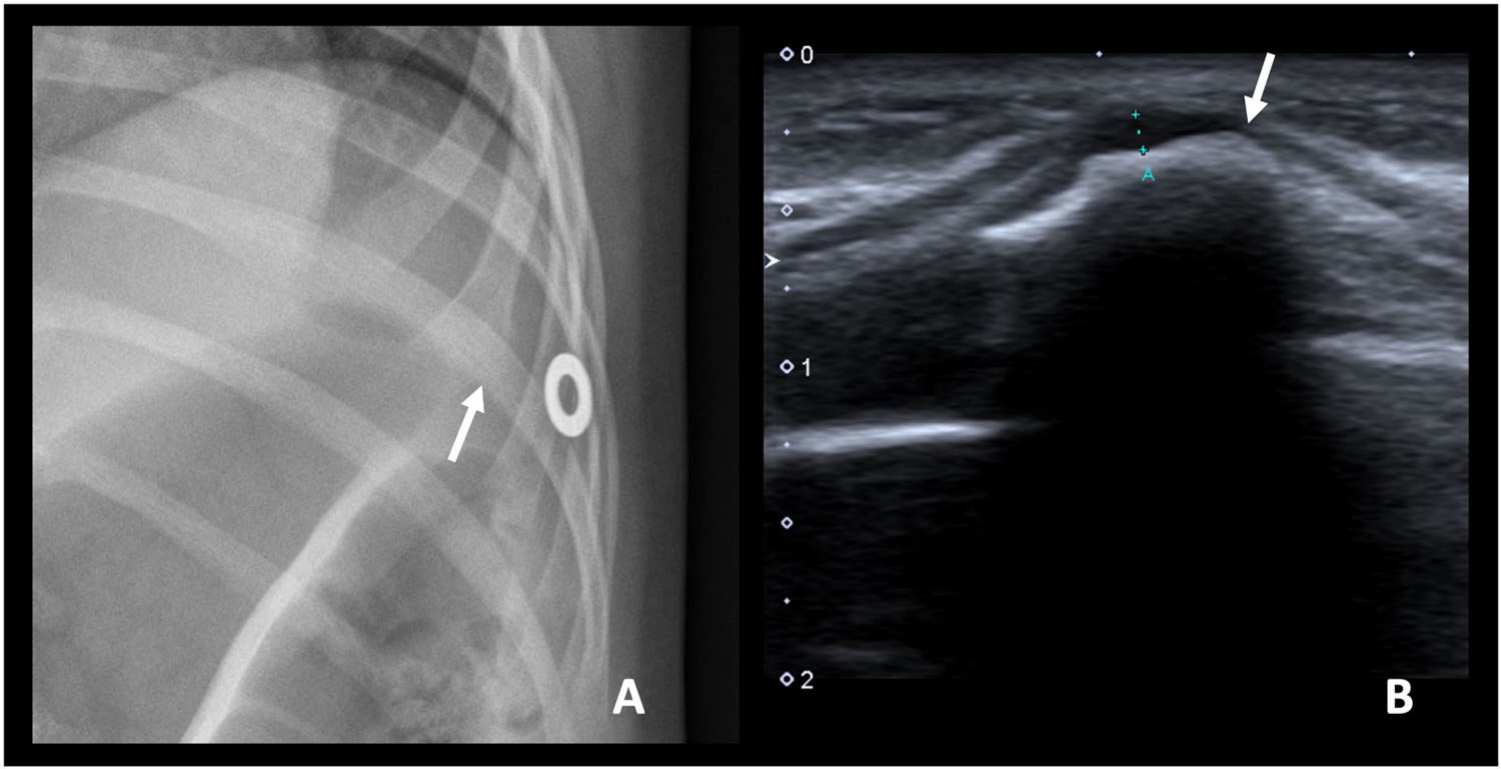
An 8-year-old girl diagnosed with HME that consulted for a palpable mass in the anterior chest wall. Conventional radiography of the ribs with a metallic marker (A) and ultrasound (B) revealed an osteochondroma in the anterior arch of a left rib (arrows). The cartilage cap measured 2 mm, within the normal range
